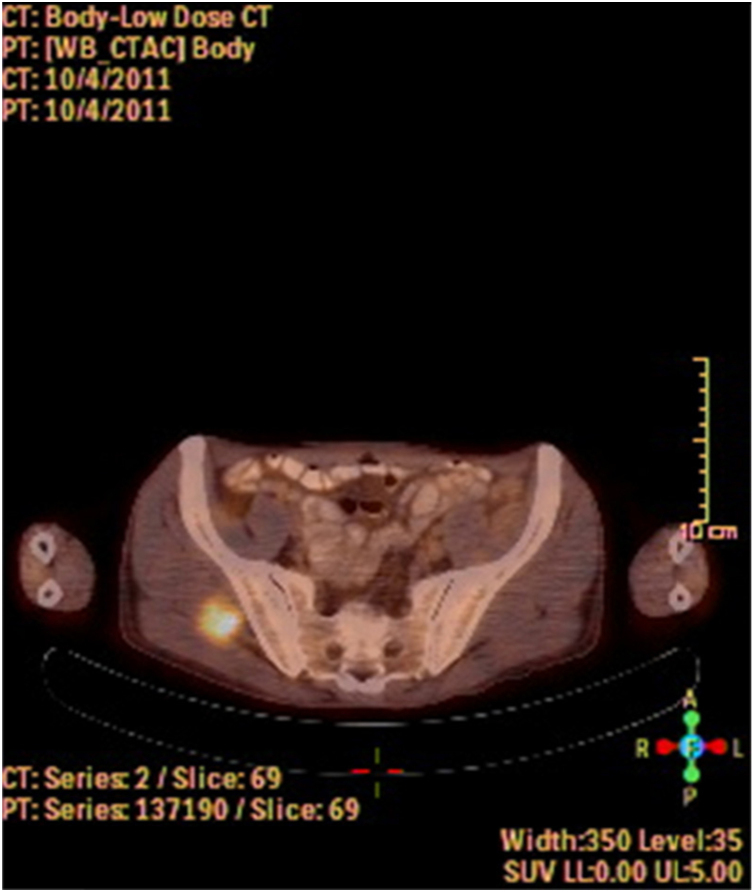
Gluteus medius metastasis from laryngeal SCC. A PET-CT image showing a dense FDG accumulation in right gluteus medius.
